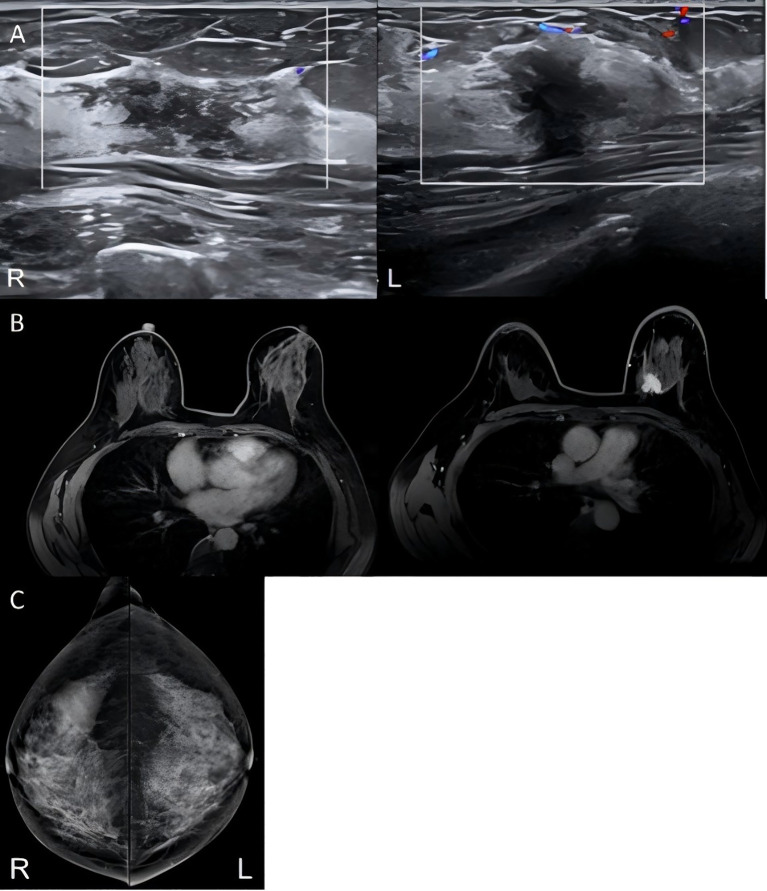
Conventional imaging findings of the patient. (A) Ultrasound: right breast shows an irregular sheet-like hypoechoic area with ill-defined borders and scant internal vascularity; left breast shows an irregular hypoechoic nodule with ill-defined borders, spiculated margins, and peripheral vascularity. (B) Contrast-enhanced MRI: left breast shows a well-defined, homogeneously enhancing mass in the upper inner quadrant; right breast is unremarkable with no abnormal enhancement. (C) Mammography: left breast shows an irregular isodense mass with spiculated margins in a heterogeneously dense background; right breast demonstrates no definite mass or suspicious calcifications.
